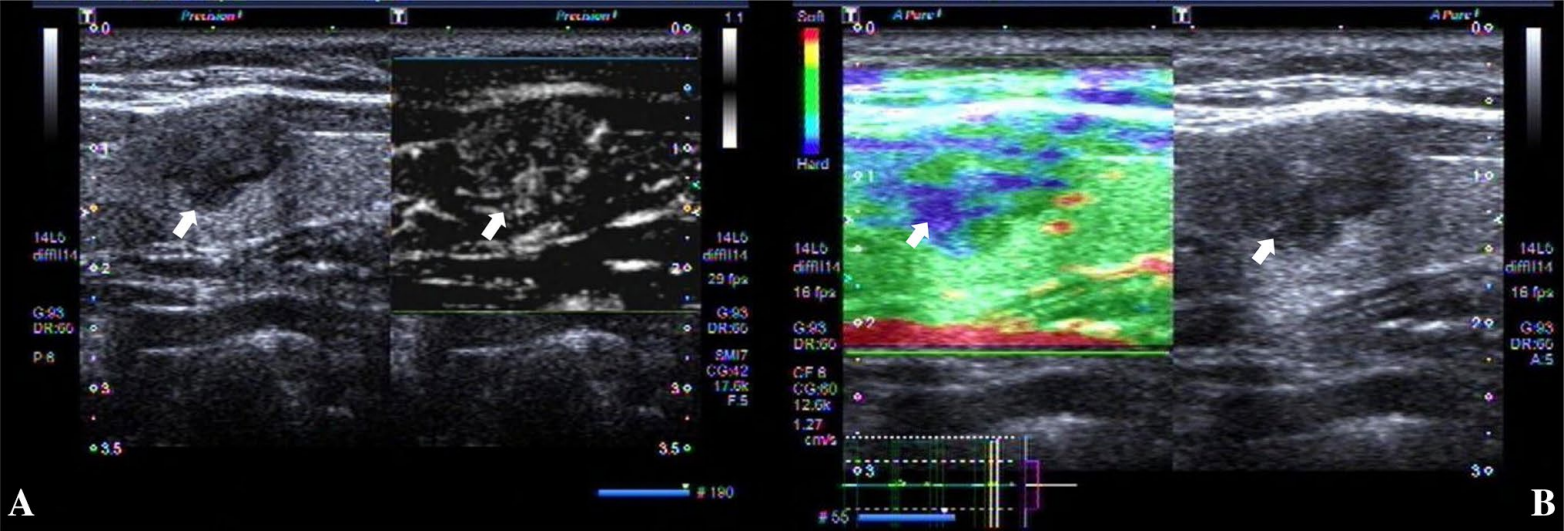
Images in a 43-year-old woman who underwent routine. A 12 mm left thyroid nodule (arrows) with solid, hypo or isoechogenicity, well defined margin, wider than tall shape, no calcification was found at conventional ultrasound and assessed as benign, ACR score of 4 and classified as TI-RADS category 4. A Type III was assigned at superb microvascular imaging. B A score of 3 was assigned at real-time elastography. This nodules was assessed as malignant at multimodal ultrasound imaging. This thyroid nodule was diagnosed as papillary thyroid carcinoma at surgery
